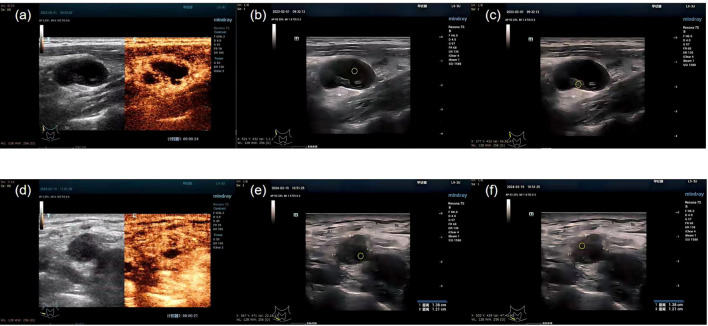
Representative grayscale and contrast-enhanced ultrasound images of necrotic cervical lymph nodes. (a–c) A patient with pathologically confirmed nasopharyngeal carcinoma cervical lymph node metastasis: (a) Contrast-enhanced ultrasound showing heterogeneous enhancement of the lymph node with a large necrotic area; (b) Grayscale ultrasound with region of interest (ROI) placed in the necrotic area for grayscale quantitative analysis; (c) ROI placed in the non-necrotic solid area of the same lymph node. (d–f) A patient with pathologically confirmed cervical tuberculous lymphadenitis: (d) Contrast-enhanced ultrasound showing ring-like enhancement of the lymph node with central necrosis; (e) Grayscale ultrasound with ROI placed in the necrotic area; (f) ROI placed in the non-necrotic solid area of the lymph node.
